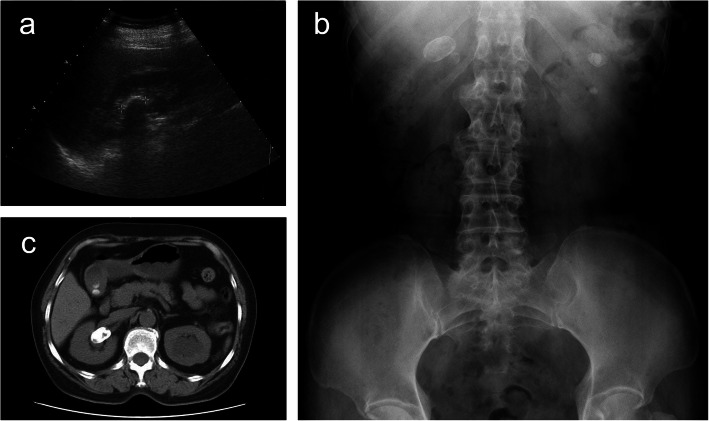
a Urinary ultrasonographic examination shows a hyperechoic focus in the right renal pelvis with an acoustic shadow. b KUB shows a large calculus in the right kidney and multiple calculi in the left kidney. c The non-contrast-enhanced CT image selected from the plain scan CTU sequence shows a calculus with a density of 508 HU and a size of 3 × 2.3 cm, with low-density lesions located in the right pelvis
